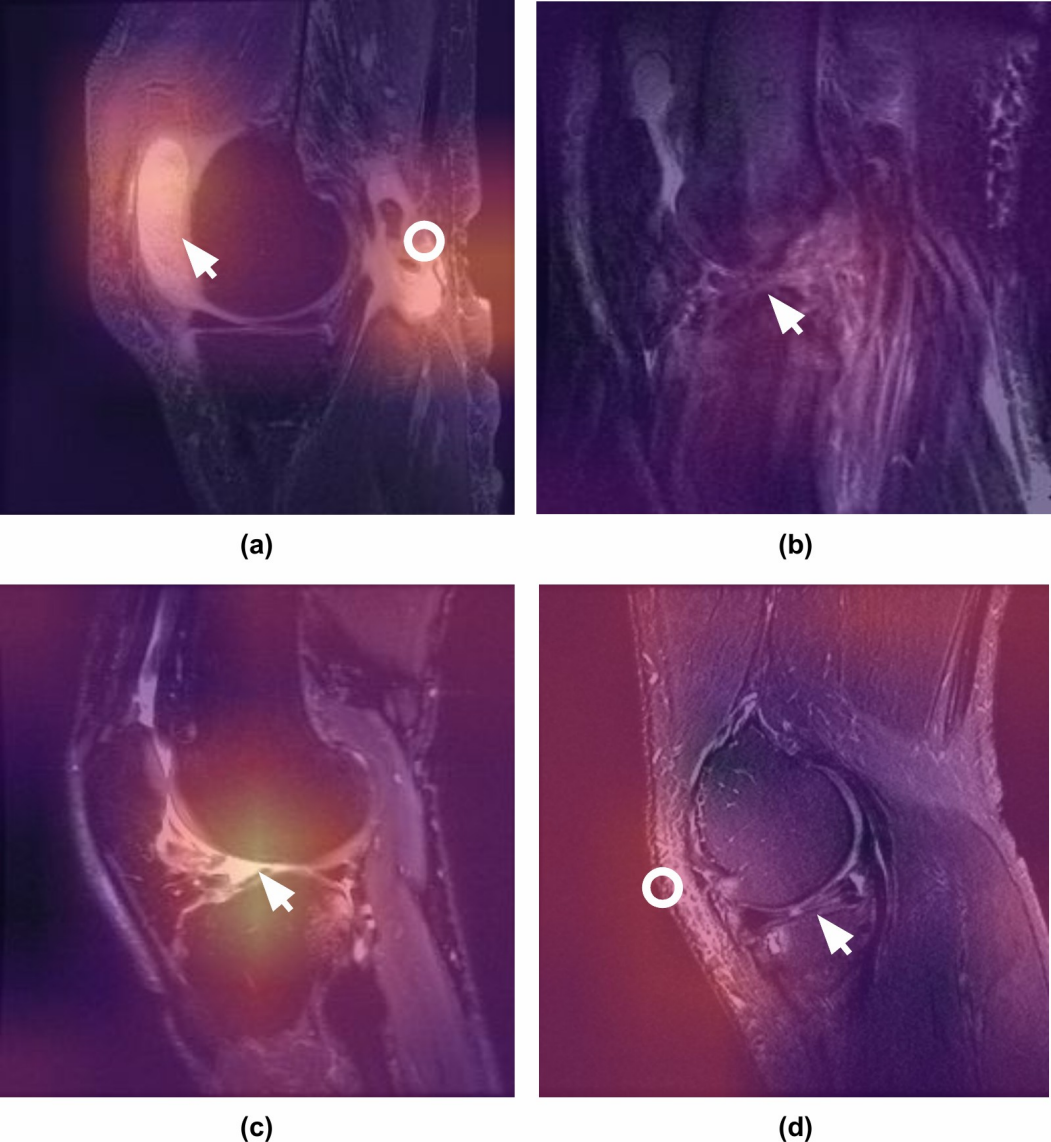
Class activation mappings for MRNet interpretation. Class activation mappings (CAMs) highlight which pixels in the images are important for the model’s classification decision. One of the board-certified musculoskeletal radiologists annotated the images (white arrows and circles) and provided the following captions. (a) Sagittal T2-weighted image of the knee demonstrating large effusion (arrow) and rupture of the gastrocnemius tendon (ring), which were correctly localized by the model and classified as abnormal. Note that the model was not specifically trained to detect these pathologies but was able to recognize the abnormalities based on the contrast with the normal knee examinations. (b) Sagittal T2-weighted image of the knee complicated by a significant motion artifact demonstrating complete anterior cruciate ligament (ACL) tear (arrow), which was correctly classified and localized by the model. Because we hoped to best approximate the clinical practice reality—in which the prevalence of artifacts (i.e. motion, metallic) and other technical noise disrupts interpretation of knee MRI—we did not exclude noisy cases from the training or validation data. (c) Sagittal T2-weighted image of the knee demonstrating complete disruption of the ACL, which was correctly identified by the model as abnormal and classified as ACL tear. The CAM indicates the focus of the model at the abnormal attachment of the ACL (arrow). (d) Sagittal T2-weighted image of the knee demonstrating a complex tear involving the posterior horn of the lateral meniscus (arrow). While the model did classify this examination as abnormal, the CAM indicates that the increased subcutaneous signal (ring) in the anterior/lateral soft tissues contributed to the decision but the meniscal tear did not.
